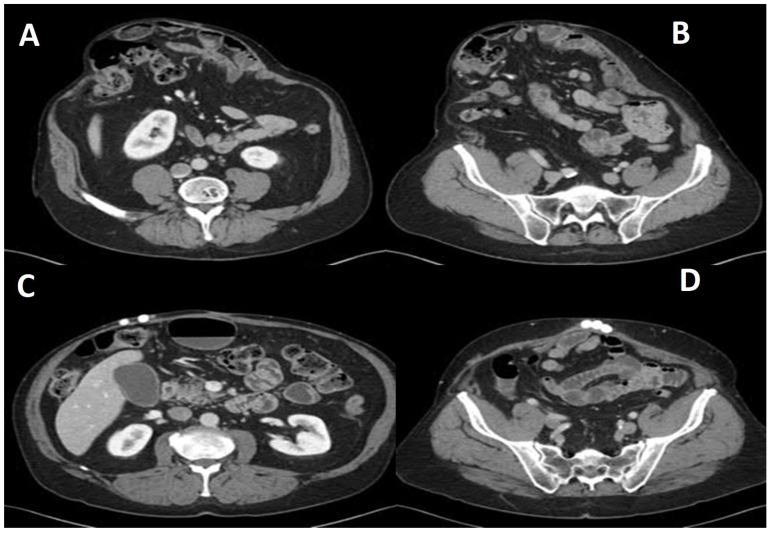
(A,B) Axial section of the preoperative CT scan shows a large ventral hernia with loss of domain. (C,D) Axial section of the postoperative CT scan shows hernia repair with antibiotic beads in situ.
